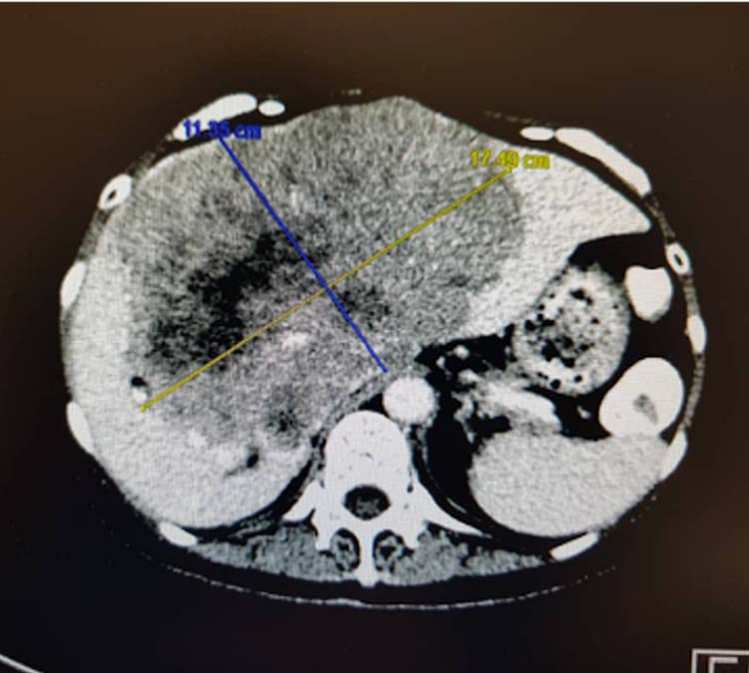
Axial CT scan showing liver metastasis at diagnosis.
